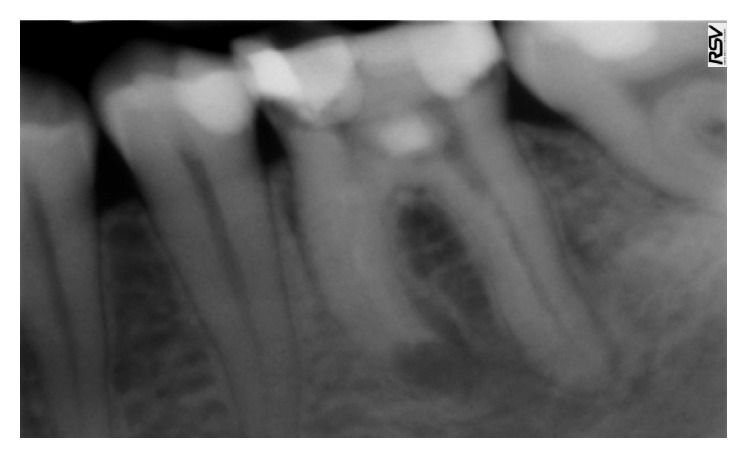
Radiograph with temporary therapeutic agent.
